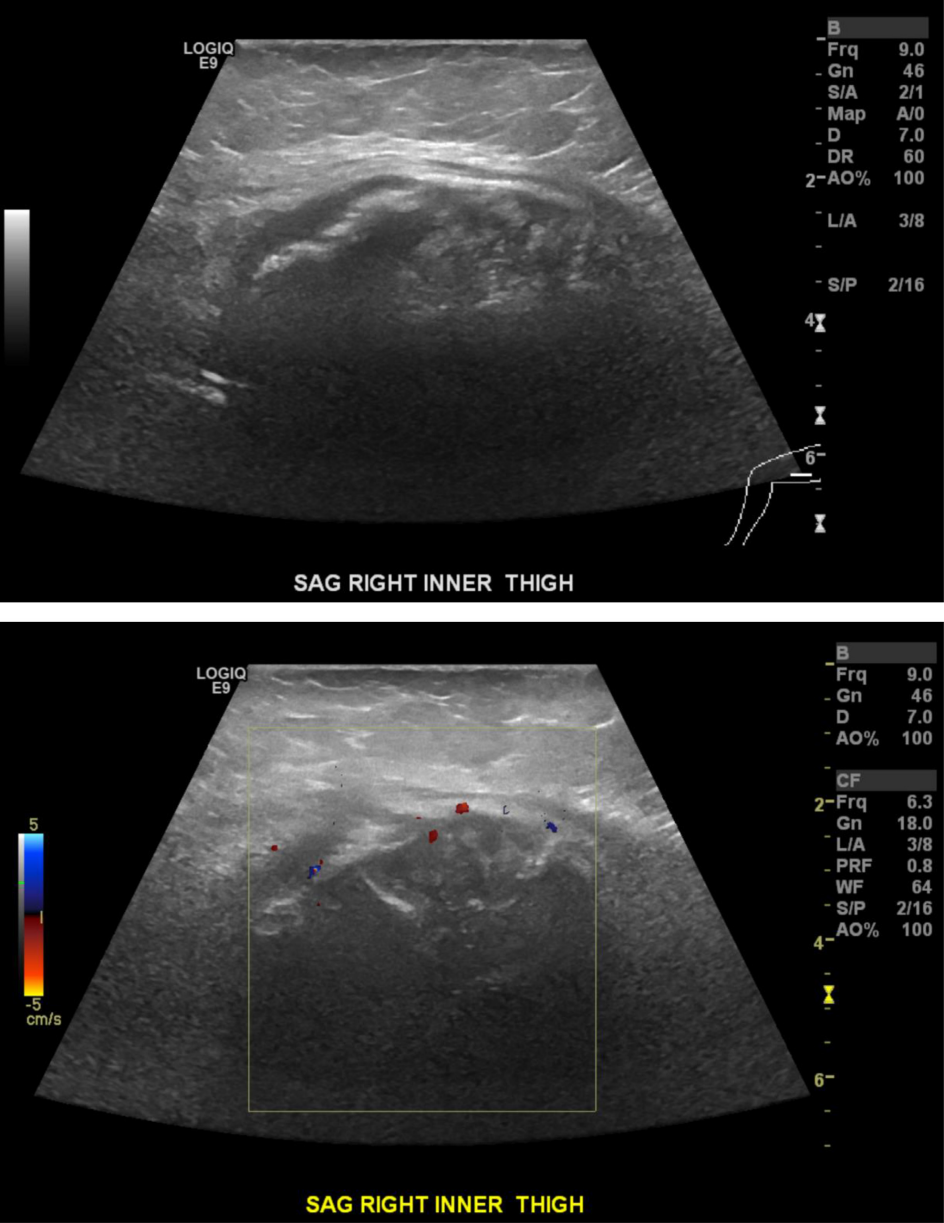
Targeted sonographic assessment of the right inner thigh showed a hypoechoic mass with dense calcification and significant posterior acoustic shadowing.
